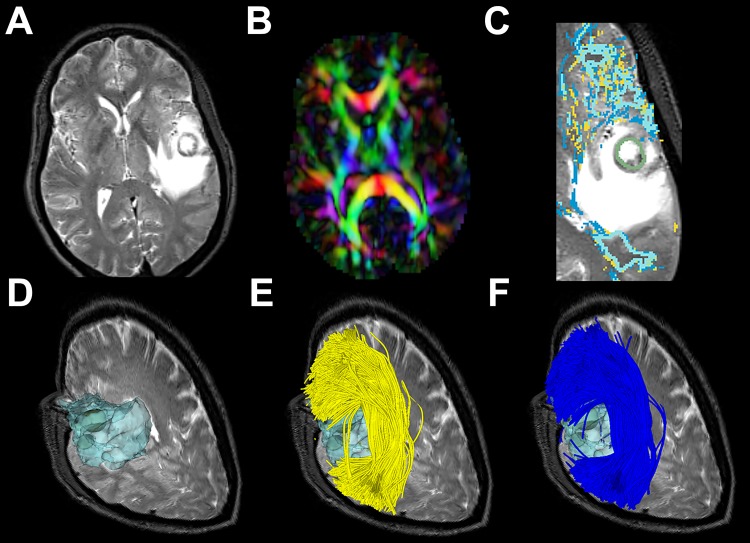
Images from P22 with a left frontal-parietal lesion. (a) T2-weighted image shows a temporal tumor with extensive edema around the lesion. (b) Directionally encoded color FA map shows reduced anisotropy in AF. (c) Magnified axial view of a T2-weighted image with overlaid label maps showing fibers reconstructed by the UKFt (yellow) and UKFt+FW (dark blue) methods and where they overlap (light blue) near the tumor (green outlined). (d) The reconstructed edematous area (transparent blue surface model) near the tumor (green model). (e) The AF using the UKFt method displayed in yellow. (f) The AF using the UKFt+FW method displayed in blue.
